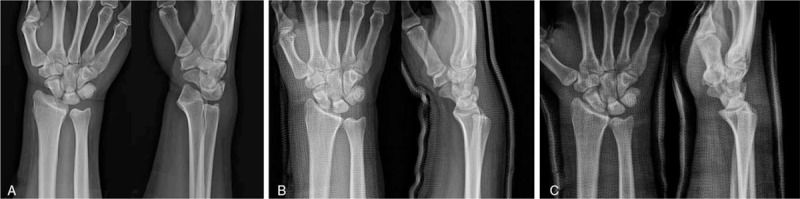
Preoperative plain radiographs. Carpal-ulnar distance ratio (CUDR) was used for measuring ulnar translation of the carpus. (A) Initial (CUDR = 0.16), (B) closed reduction (CUDR = 0.24), and (C) 3 weeks after the injury (CUDR = 0.16).
